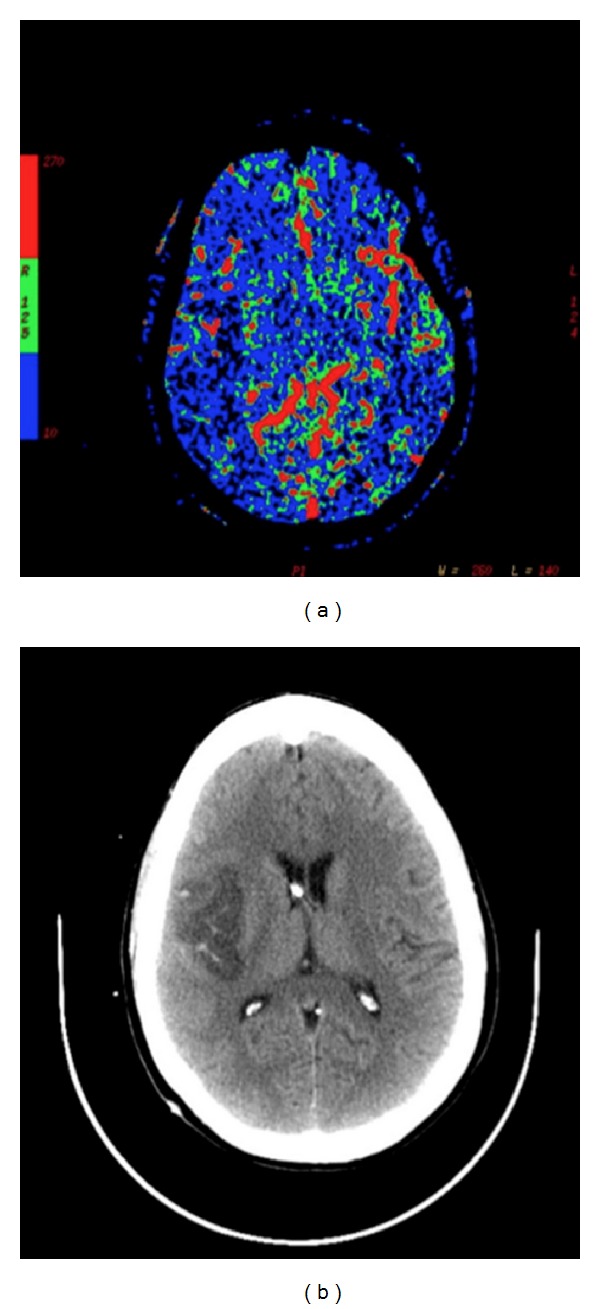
CT Perfusion. Decreased perfusion secondary to vasospasm in the left middle cerebral artery territory on CTP (a). Corresponding ischemia is noted on CT scan (b).
